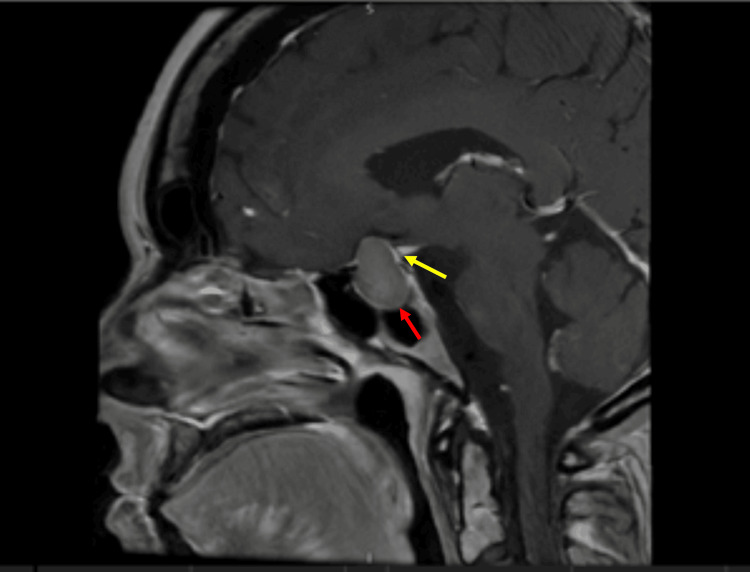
Contrast-enhanced sagittal view of the MRI scan eight months after initial presentation. The size of the tumour (red arrow) was unchanged, and it continued to remain in contact with the optic chiasm (yellow arrow).
